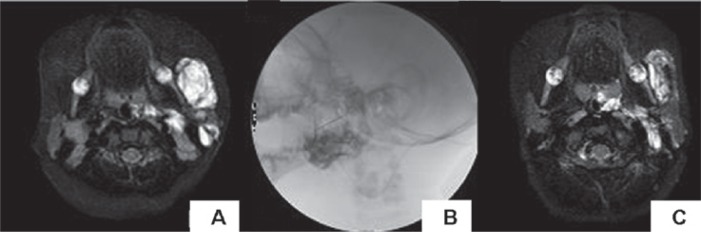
The patient in these images was 8 years old. (A) Venous malformation was located in the left tempus; (B) Percutaneous interventional sclerosing therapy was guided by MRI. During surgery 5 mg bleomycin, 2.5 ml iohexol and 2.5 ml ultra-liquefied lipiodol suspension were used; the patient was treated twice. (C) The patient was reviewed two months after surgery and the tumors were reduced in size.
